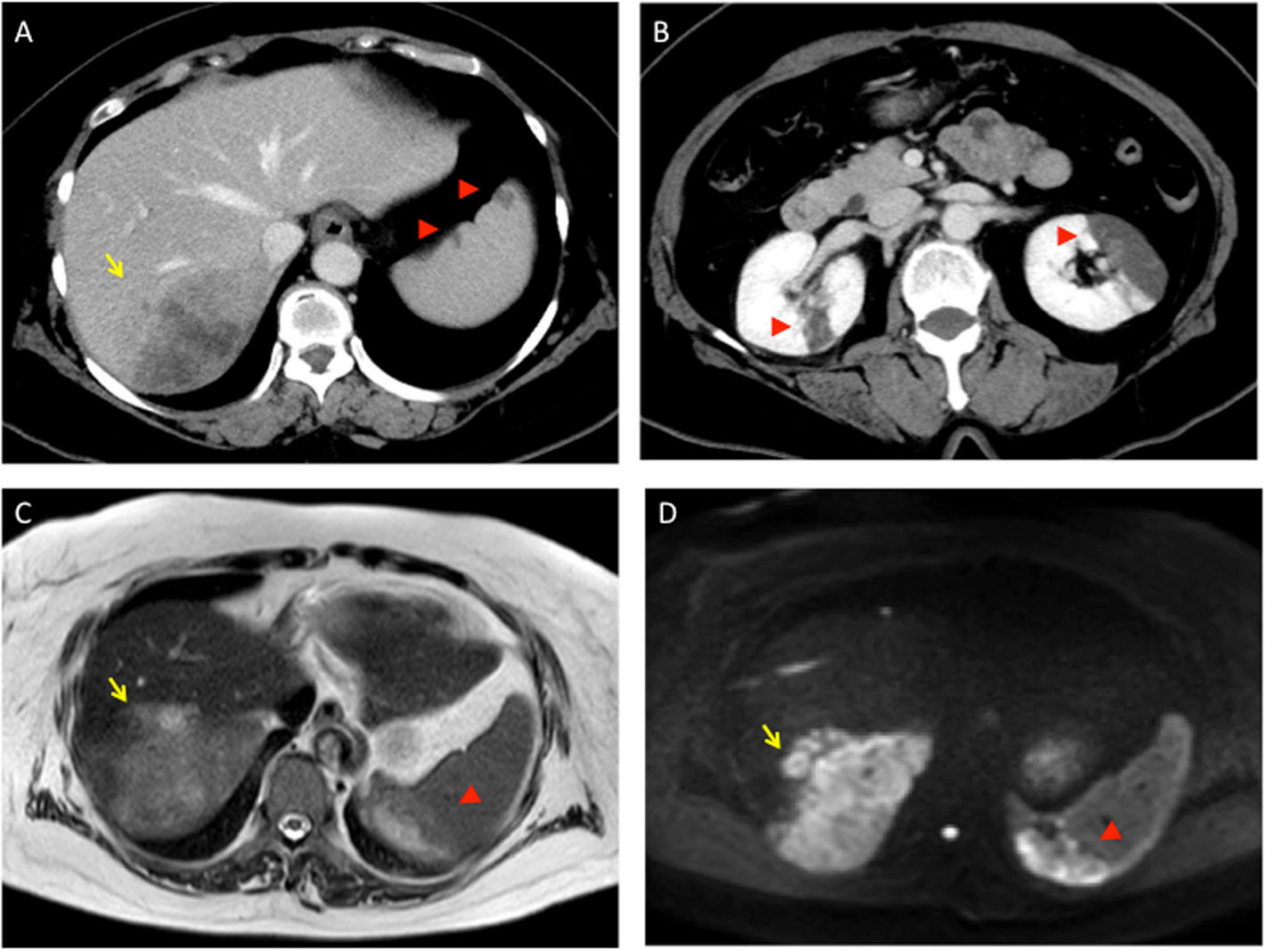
Abdominal enhanced CT and MRI findings. a CT showing a tumor in the posterior segment of the right hepatic lobe (arrow) and splenic infarctions (arrowhead). b CT showing renal infarctions in both kidneys (arrowhead). c, d MRI showing a tumor in the posterior segment of the right hepatic lobe (arrow) and splenic infarctions (arrowhead). CT, computed tomography; MRI, magnetic resonance imaging
